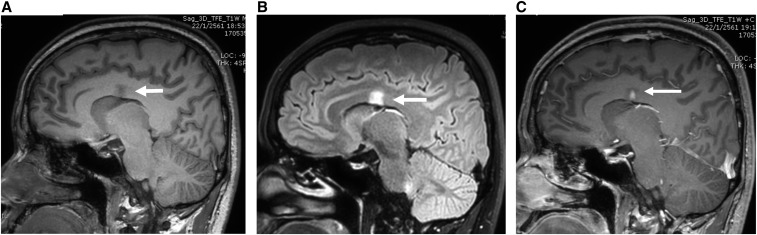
Magnetic resonance imaging of the brain showed a focal lesion at the body of the corpus callosum, which exhibited low signal intensity on sagittal T1-weighted images (A), high signal intensity on fluid-attenuated inversion recovery images (B), and nodular enhancement after administration of gadolinium (C).
